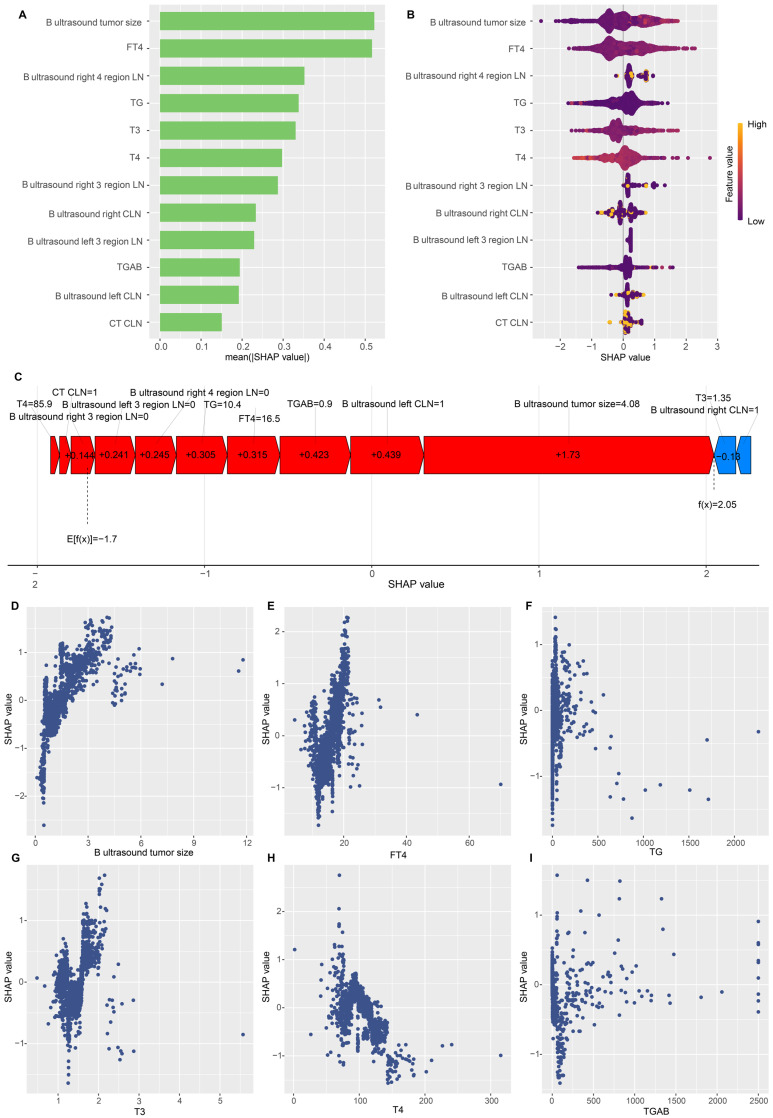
Global and local model explanation by the SHAP method. (A) Summary plot showed the 12 features ranking by mean absolute SHAP values. (B) Each variable name is shown on the left-hand side with the variable with the greatest contribution listed at the top. To the right of the variables, there are colored lines, which are individual points that correspond to observations in the population. A higher value for the variable is represented in yellow, while a lower value for the variable will be shown in purple. A value farther to the right (ie, a higher SHAP value) indicates that the variable is contributed to a prediction of a positive target, such as DLNM. (C) An example of risk factor analysis for a patient with PTC which represented the individual PTC towards the “DLNM” class. (D-I) One-way SHAP dependence plot of the 6 important predictors (continuous variable). (D) B ultrasound tumor size. (E) FT4. (F) TG. (G) T3. (H) T4. (I) TGAB. Each dependence plot shows how a single feature affects the output of the prediction model, and each dot represents a single patient. Specifically, the values of the predictor are represented by the x-axis, and its SHAP values are represented by the y-axis. To interpret these plots, for example, in (D), patients with higher tumor size (as x-axis increased) were associated with a higher SHAP value, which indicated a higher likelihood of DLNM (y-axis also increased).
